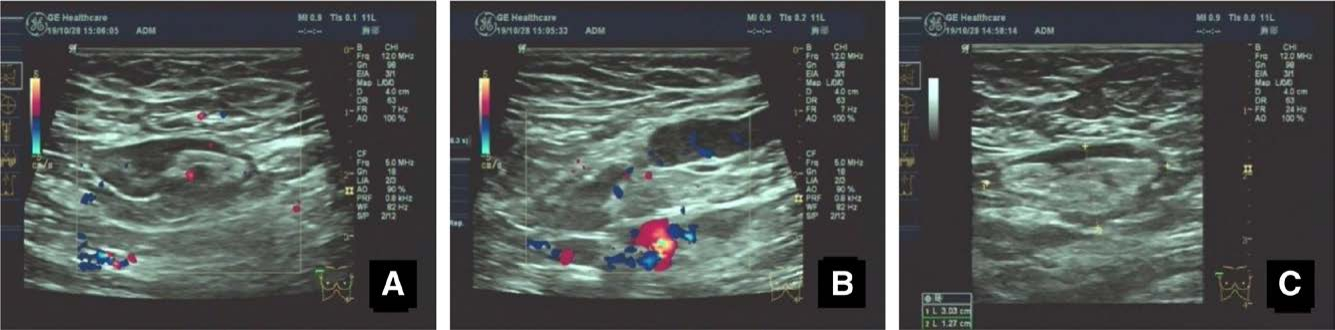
Doppler and ultrasound imaging of axillary lymph nodes. (A) Doppler blood flow image of the lymph nodes in the left axilla. (B) Doppler blood flow image of the lymph nodes in the right axilla. (C) Ultrasound image of the lymph nodes in the left axilla.
